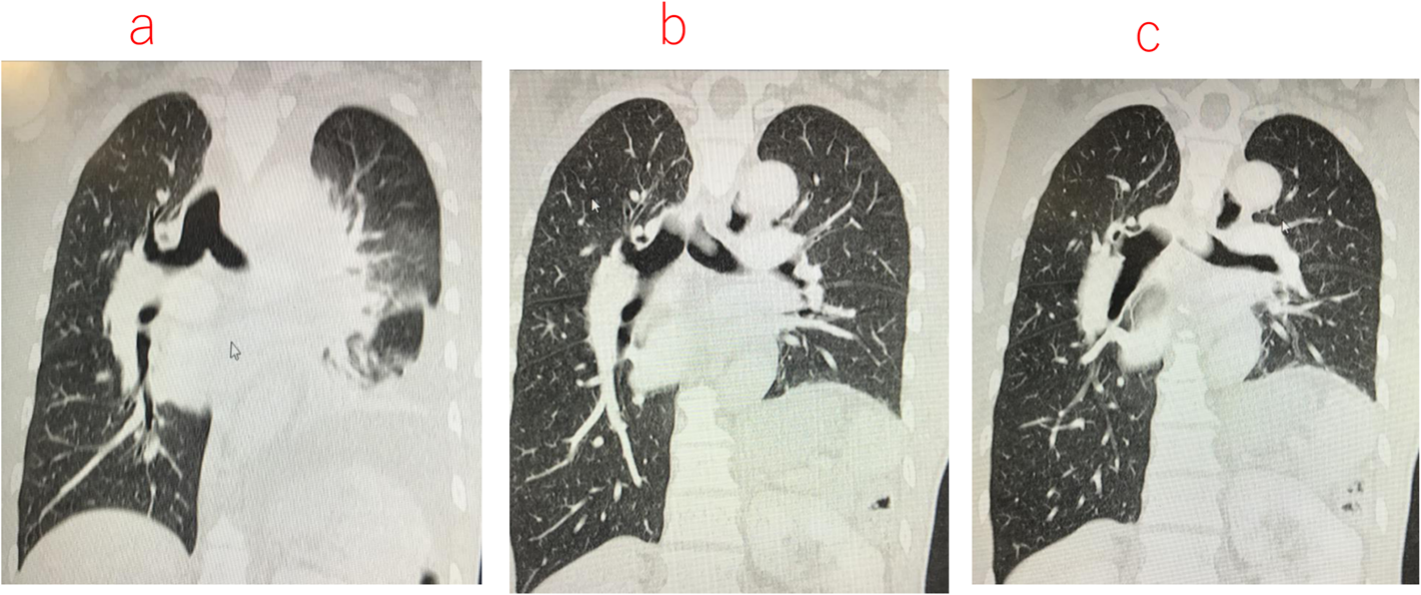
a–c CT scan showing the deformity of the left and right main bronchus. Collapsed left main bronchus due to a giant anterior mediastinal tumor (GAMT)
